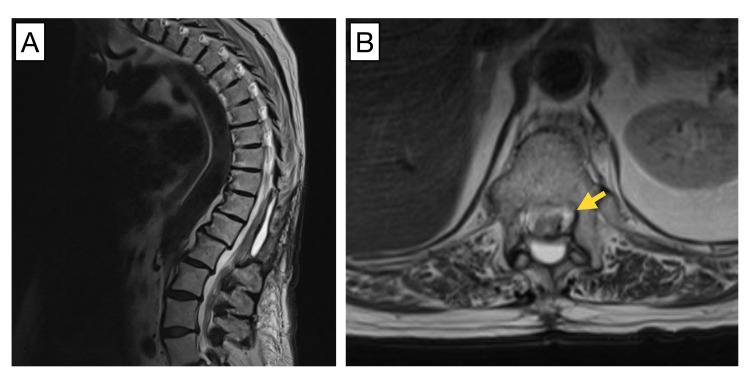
Repeat MRI at three months follow-up. Follow-up MRI showed a stable nodular focus of enhancement at the T11-12 level extending into the proximal left neural foramen (yellow arrow). Sagittal (A) and transverse (B) images are shown.
